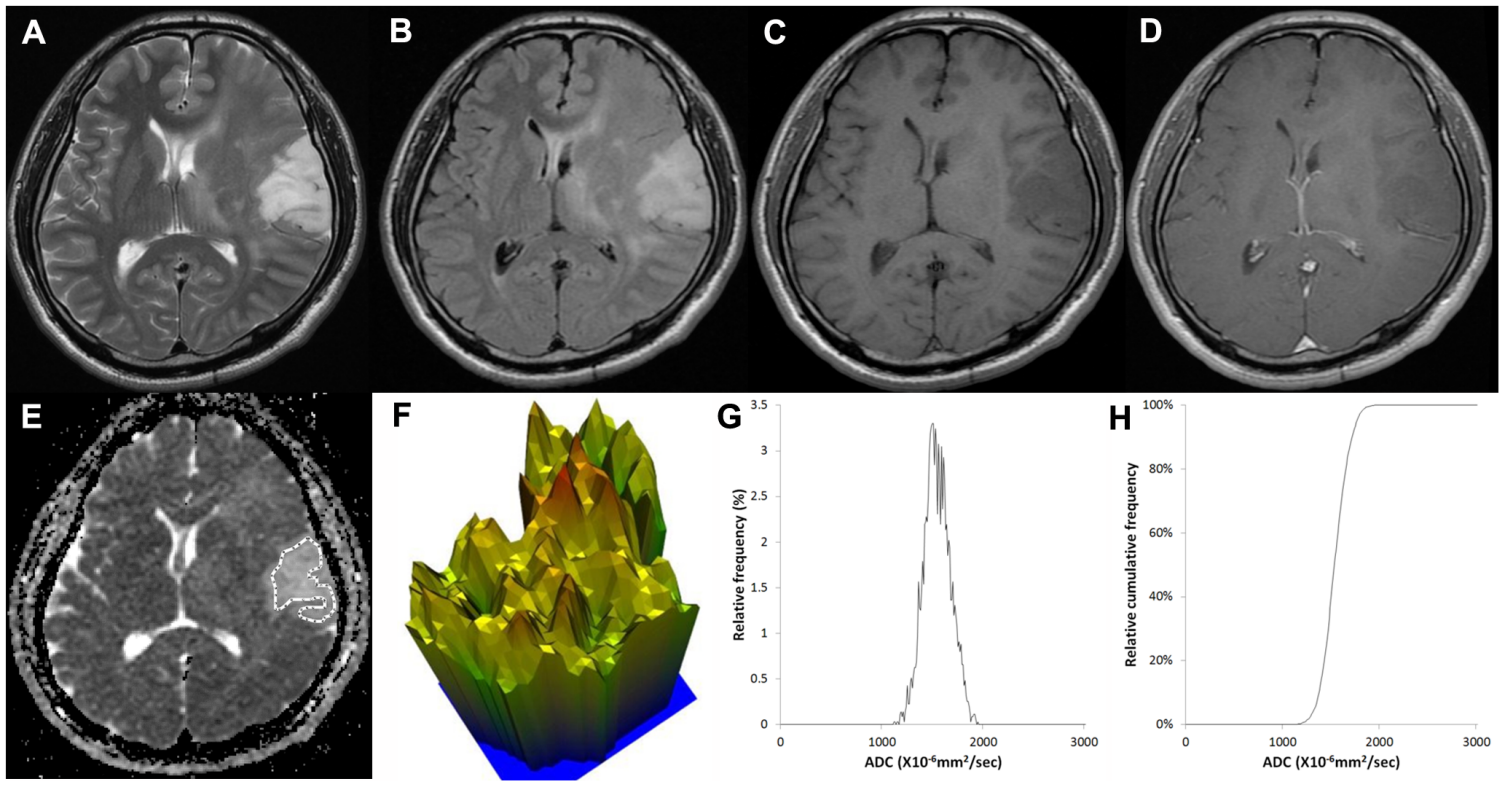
Images of a 43-year-old male with a grade II astrocytoma. (A) T2-weighted image, (B) T2 FLAIR image, (C) T1-weighted image, (D) contrast-enhanced T1-weighted image, (E) ADC map with ROI placement, with the corresponding (E) 3-D height map of the ADC signal intensity, (G) histogram of ADC and (F) cumulative ADC histogram. The entropy value of ADC was 6.168.
